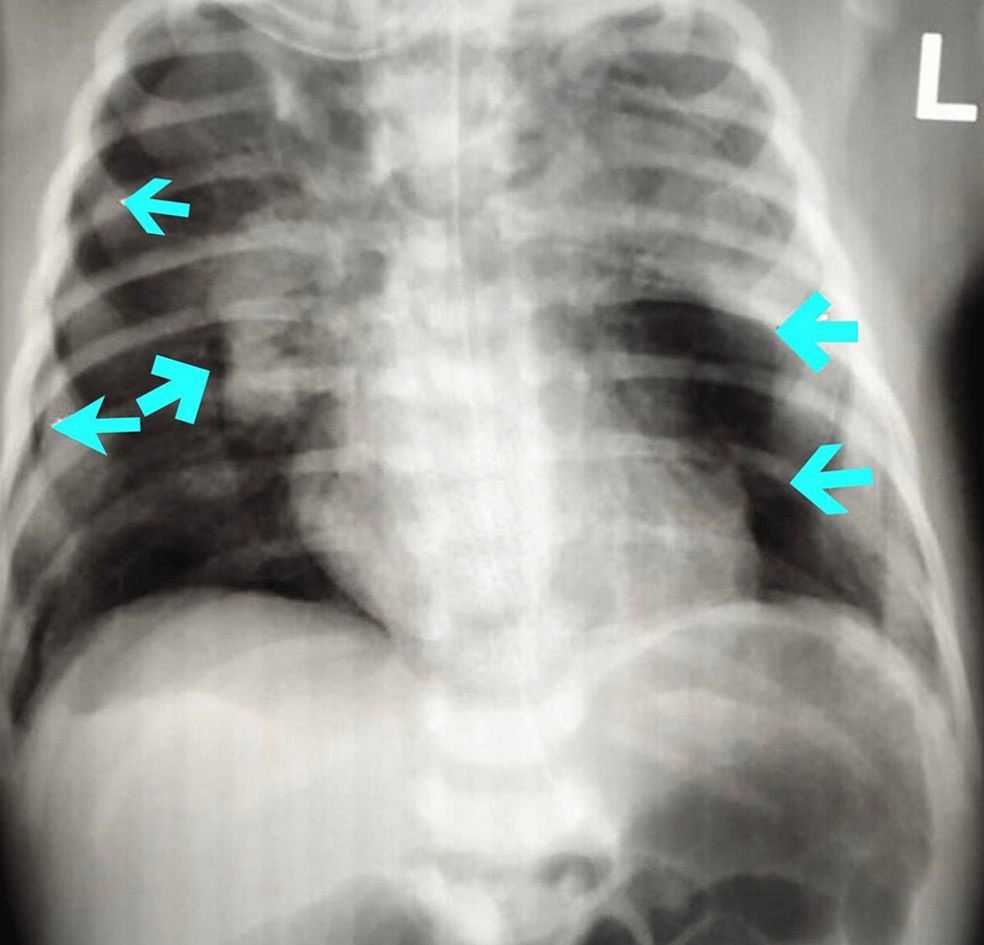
Chest X-ray, arrows demonstrating the presence of the large elliptical-shaped lucent area which is noted at the left pericardial region, causing separation and elevation of the thymic shadow, concerning pneumothorax and pneumomediastinum.
